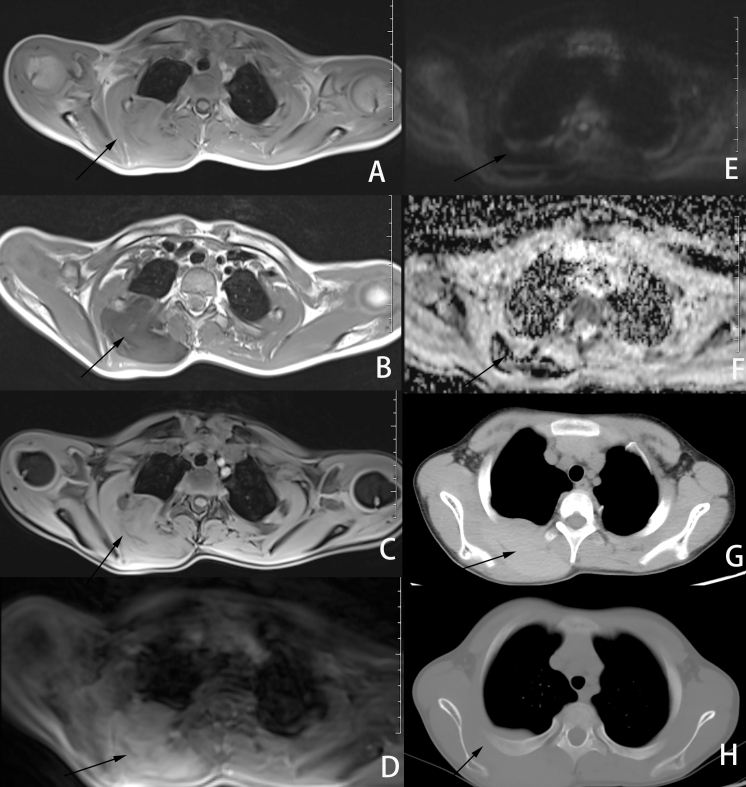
The soft tissue lesion on the right back involved intercostal muscles (A–G). The adjacent rib was thickened (F). No obvious signal change was noted after fat pressing on *T*_1_WI (C). The lesion showed hypointense signal on *T*_1_WI and hypointense signal on *T*_2_WI (A–B). The lesions showed hyperintensity on FS-*T*_1_WI after the contrast agent was administered (D). The masses show hypointense signals on DWI and ADC maps (E–F). The mass lesion showed slightly high density on CT (G). ADC, apparent diffusion coefficient; DWI, diffusion-weighted imaging; FS, fat saturated; *T*_1_WI, *T*_1_ weighted imaging; *T*_2_WI; *T*_2_ weighted imaging.
